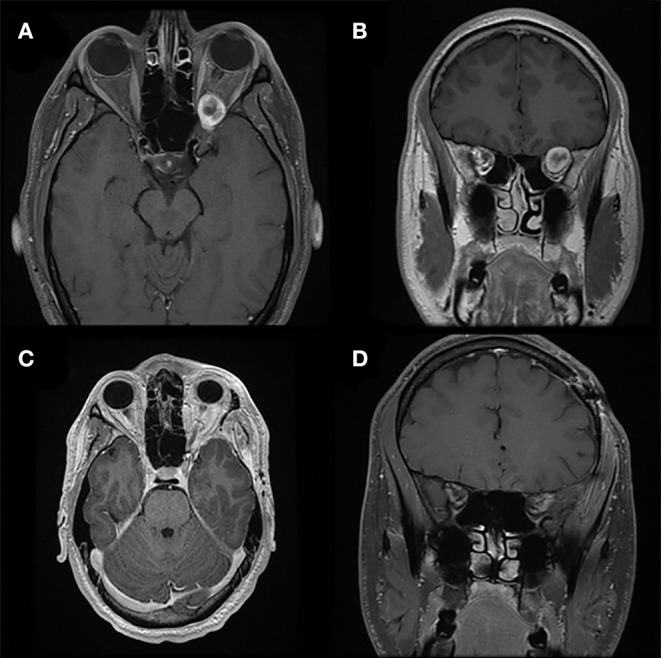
Pterional approach. Preoperative T1-weighted axial (A) and coronal (B) gadolinium-enhanced MRI of a patient with a heterogeneously enhancing lesion of the left orbital apex causing compression of the optic nerve and nerves exiting the superior orbital fissure. The location and larger size of the lesion requires a pterional cranio-orbital approach by a multidisciplinary team to remove the sphenoid wing and expose the superior and lateral aspects of the orbit and orbital apex. Postoperative T1-weighted axial (C) and coronal (D) gadolinium-enhanced MRI demonstrate complete resection of this lesion associated with resolution of symptoms.
